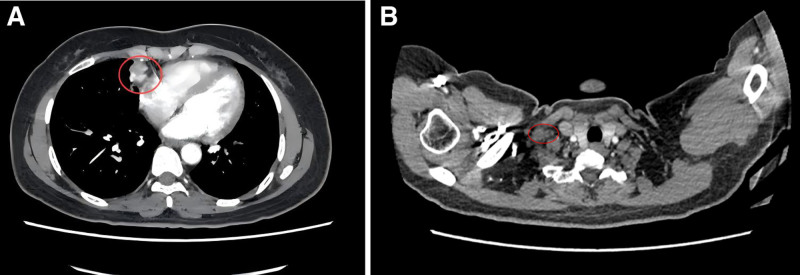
Chest CT follow-up on September 9, 2020. (A) Slight enlargement of the right lung lesion compared to June 11; (B) enlargement of the right supraclavicular lymph node. CT = computed tomography.
